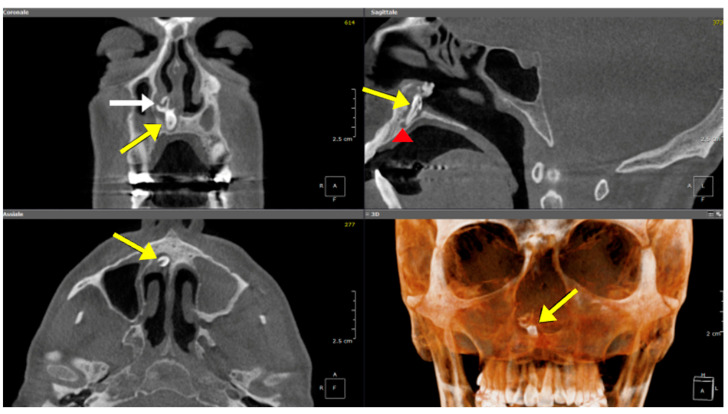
Inverted mesiodens in a 60-year-old male with unilateral nasal obstruction and blood discharge from his nose submitted to a CBCT. CBCT shows an inverted supernumerary tooth (inverted mesiodens) erupted into the right nasal cavity. The inverted mesiodens (yellow arrow), was carious with a periapical lesion (red arrowhead) and its crown was surrounded by reactive tissue with a rhinolith (white arrow).
